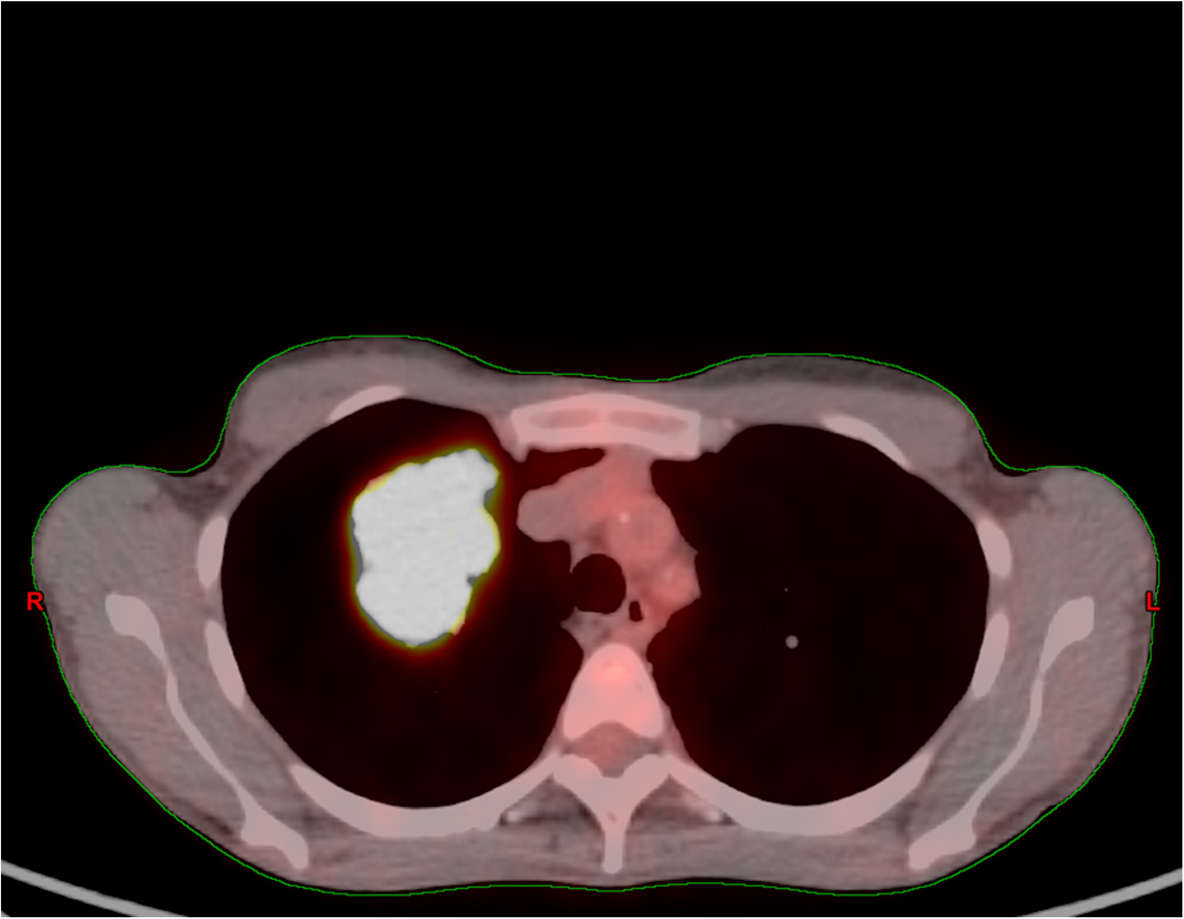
An axial section of the treatment planning computed tomography scan fused with positron emission tomography. A right upper lobe lung mass can be seen.
